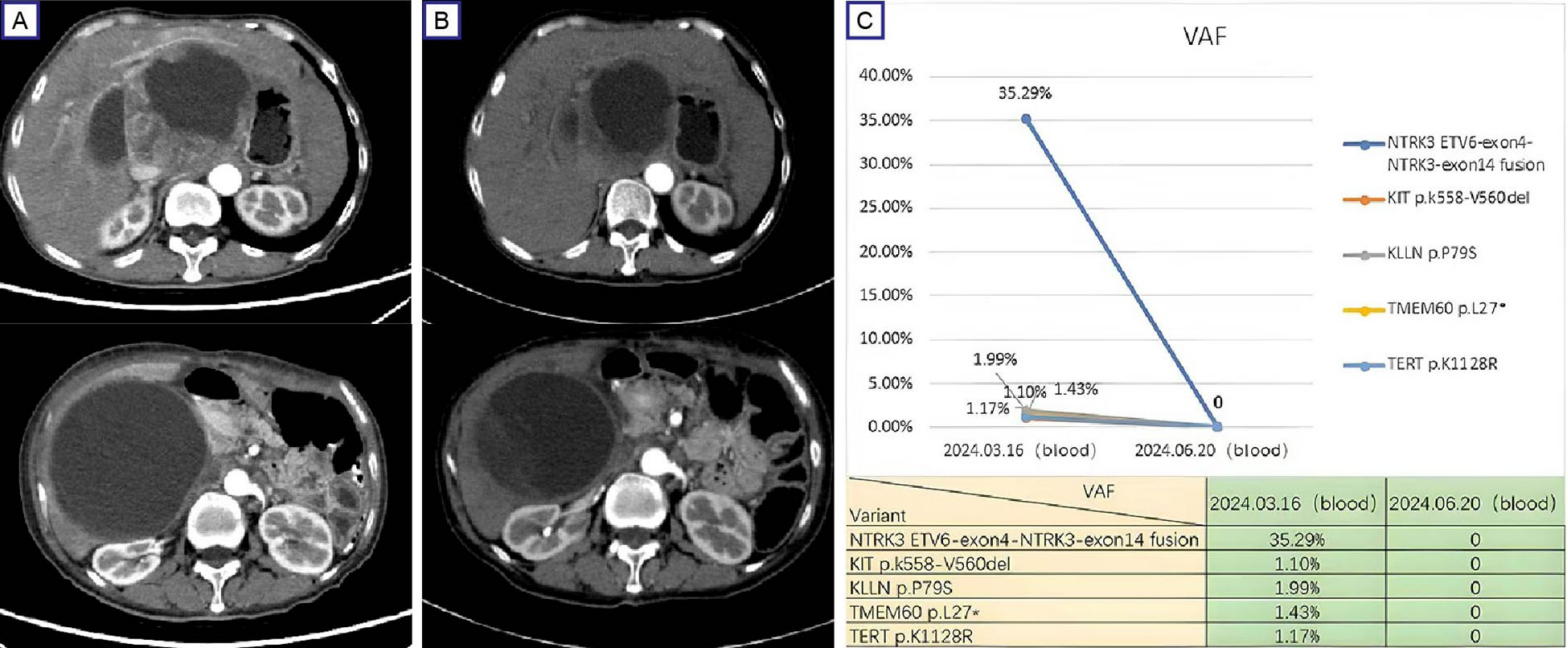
Therapeutic response and mutation dynamics in progressive GIST. (A) Pre-treatment contrast-enhanced CT (March 28, 2024) demonstrates a dominant cystic-solid mass (12.6 cm×10.8 cm, arrows) in the hepatogastric space with IVC compression; (B) Post-treatment (entrectinib) contrast-enhanced CT (April 25, 2024) shows partial response (RECIST 1.1) with 27% tumor reduction (9.6 cm × 8.4 cm) and improved IVC compression. (C) ctDNA profiling reveals clearance of *KIT* exon 11 deletion (p.K558_V560del) and *ETV6:NTRK3* fusion (ETV6 exon4:NTRK3 exon14) following entrectinib therapy. IVC, inferior vena cava.
